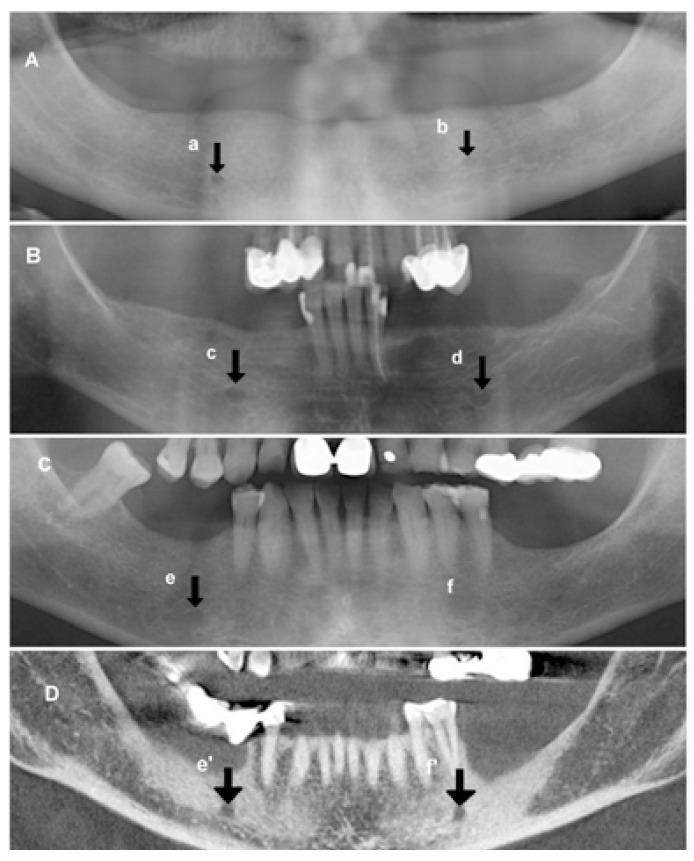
Mental foramen on panoramic radiographs (A,B,C): continuous (a), separated (c,d), diffuse (b,e), and unidentified (f) (Yosue and Brooks). Mental foramen on cone beam CT (D): The left mental foramen (f’) is visualized using para-panoramic reconstruction corresponding to patient C).
